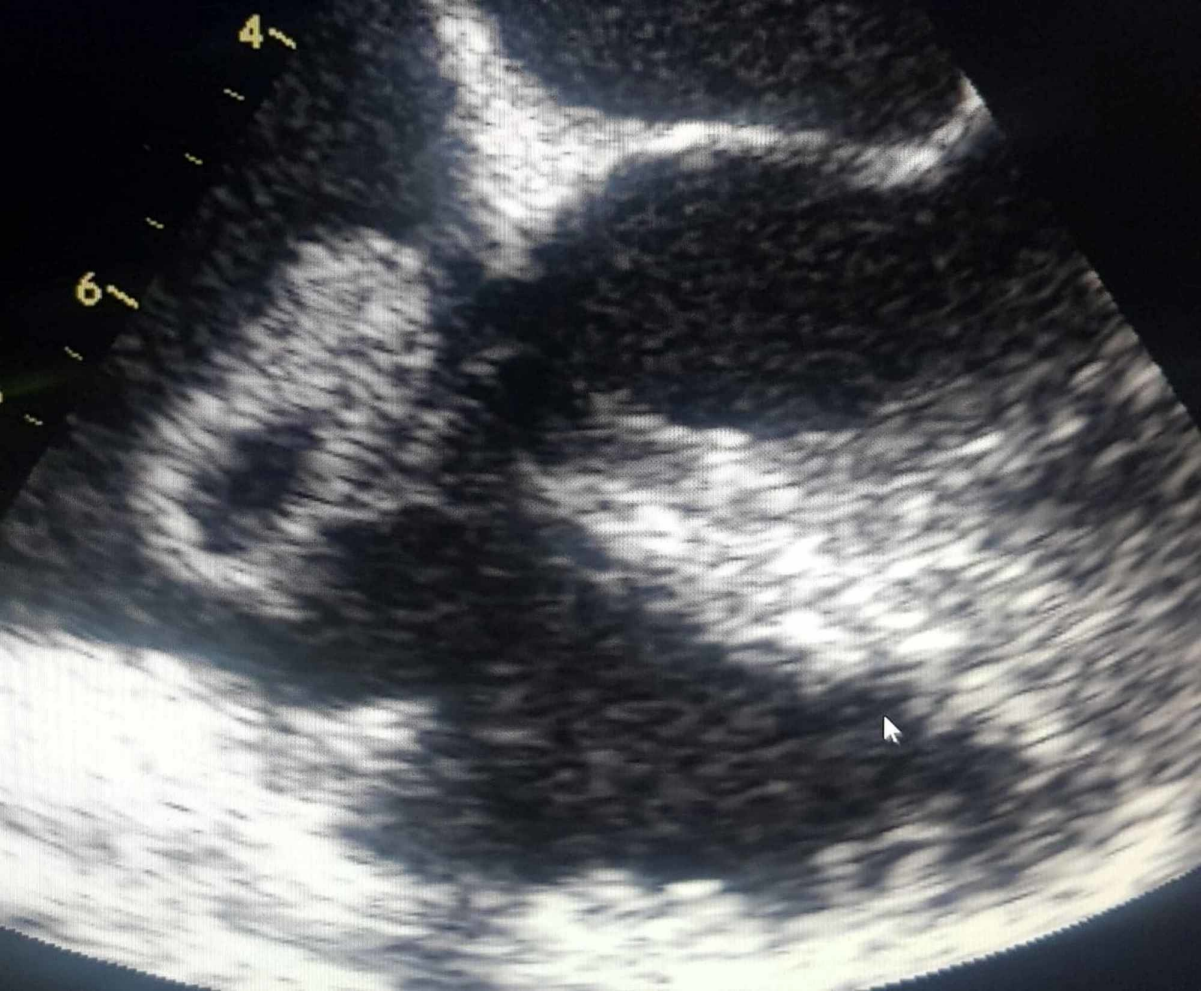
TEE showing large vegetation of tricuspid valve with central liquefaction. TEE, transesophageal echocardiography
